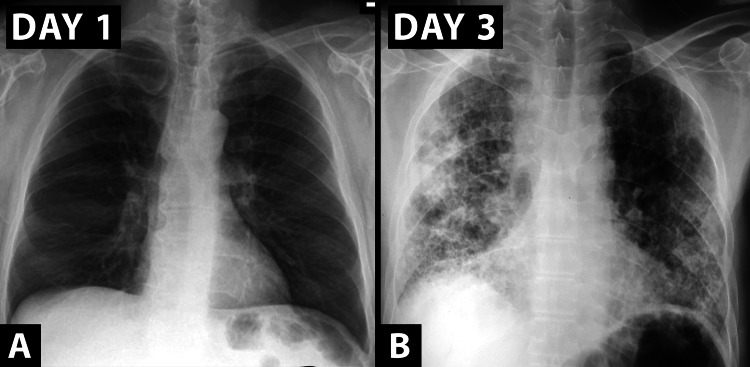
Chest radiographs showing A) normal findings at presentation, B) development of bilateral heterogenous opacities with peripheral predominance, likely alveolar hemorrhage
